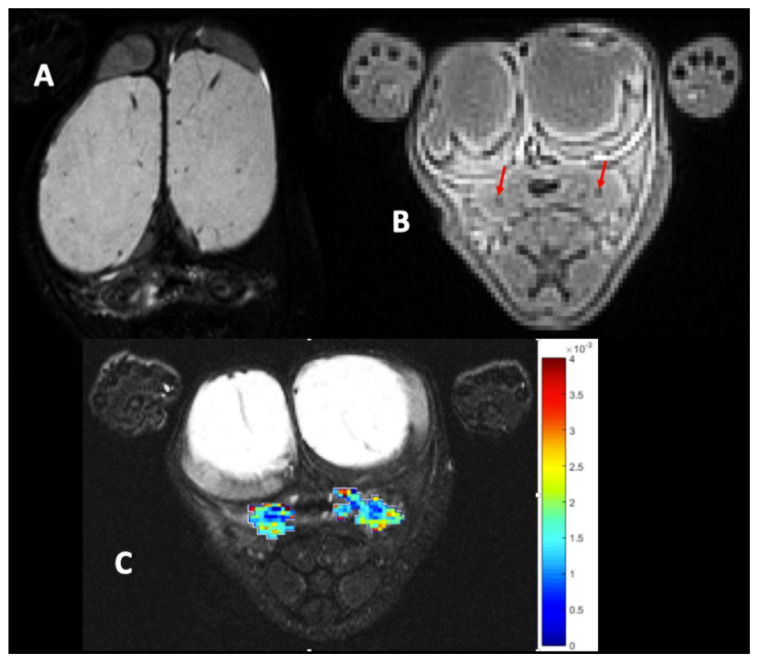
Baseline MRI. (A): T2-weighted image, axial section, hyposignal of the fistula tract, and hypersignal of peripheral inflammation. (B): Axial ultra-short echo time (UTE) images evidenced the 2 visible tracks (red arrows). (C): Inflammation is seen as a high apparent diffusion coefficient (ADC) area on the ADC map. The ADC scale is located on the right.
